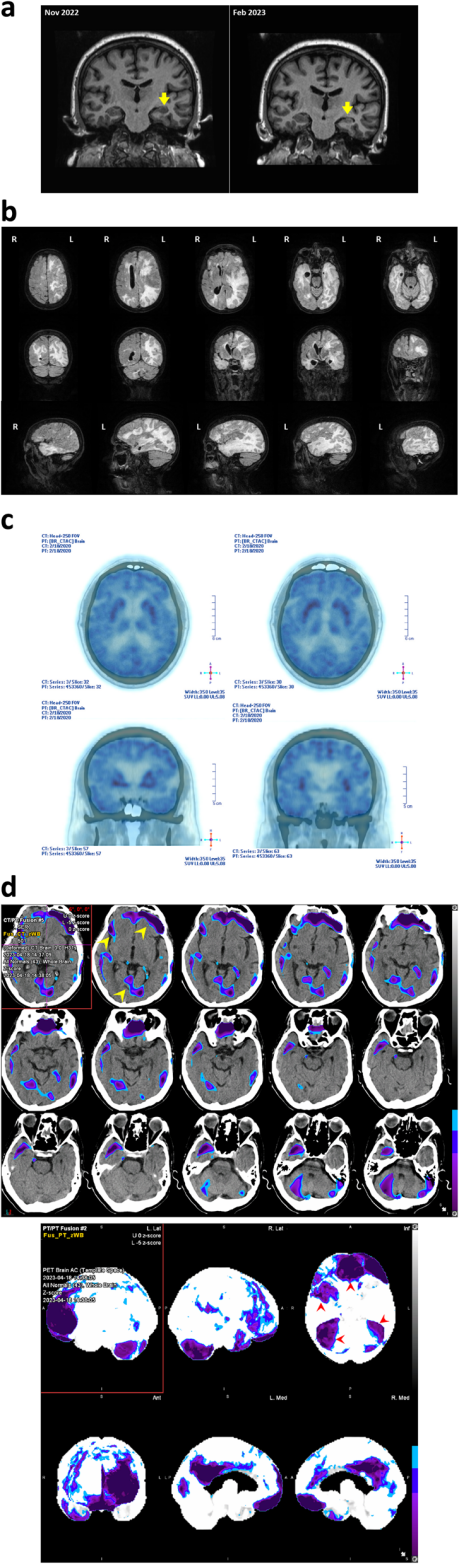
a The case #6 developed asymmetric hippocampal atrophy as seen in serial brain MRI images. b Fulminant cerebral edema seen in case #5, more in the left hemisphere. c Brain PET revealed hypermetabolism in the basal ganglion in case #2 with FBDS. d The case #8 showed bilateral hypometabolism in the frontal and temporal lobes with rapid neurocognitive decline (brain PDG-PET scan); biodistribution shows statistically significant hypometabolism with very low Z-scores (-3, shown as purple-blue pseudo-color) in the posterior cingulate gyrus, as well as frontal (left worse than right) and temporal lobes (right worse than left). Upper panel shows the spatial structural localization of the hypometabolism; lower panel shows the fusion of images with low structural resolution to highlight the affected brain regions
